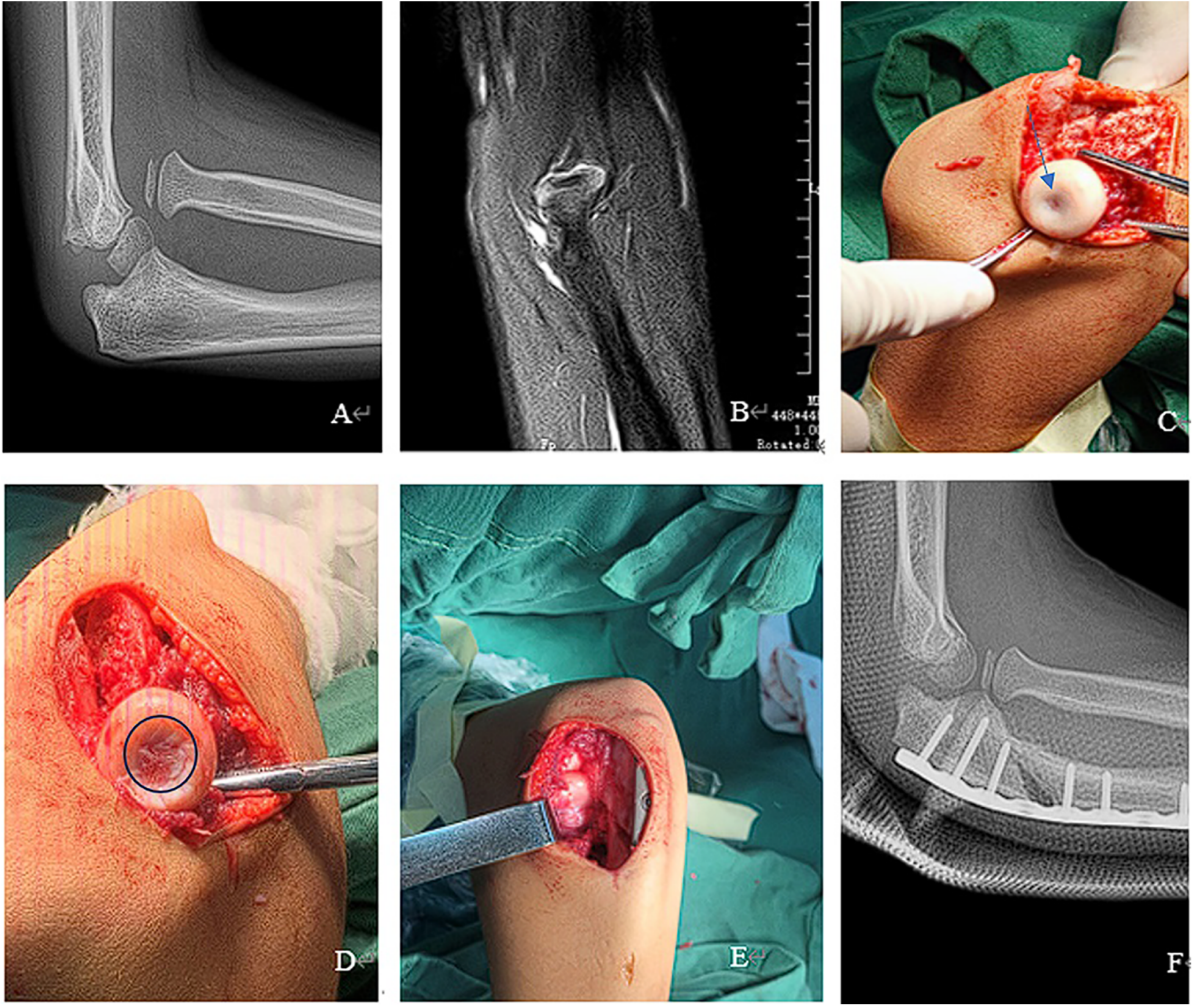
(A) flat radial head in X, (B) flat radial head in MRI, (C) flat radial head in operative, arrows display the area that needs to be scraped off, (D) scraping concave surface, circular display shows the area after scraping, (E) radiocapitellar joint plasty, (F) post-operative in X.
